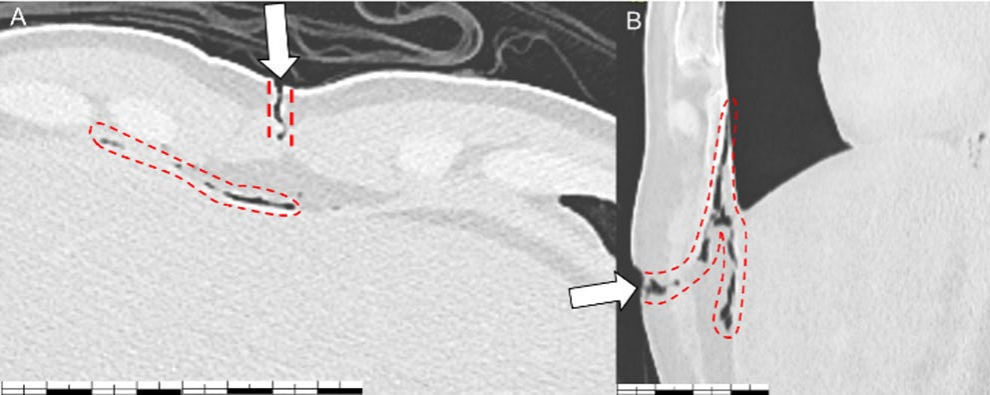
CT scan of the wound (horizontal section A and sagittal section B) white arrow indicates the wound, while the red dotted line highlights the emphysema localized in the subcutaneous soft tissues and mediastinum, particularly anterior to the diaphragm and pericardium
